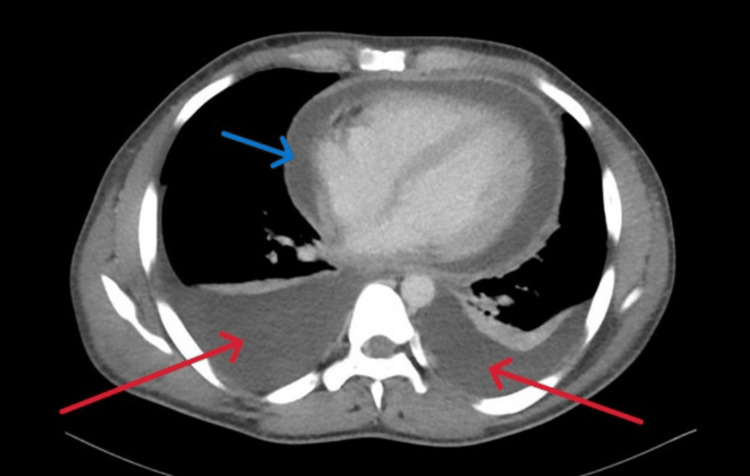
Axial CT thorax The red arrows showed bilateral pleural effusion, the blue arrow revealed cardiomegaly with pericardial effusion.
